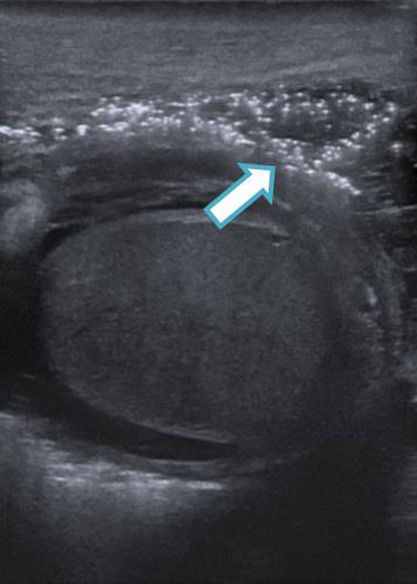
Ultrasound image demonstrating gas within the scrotum.
